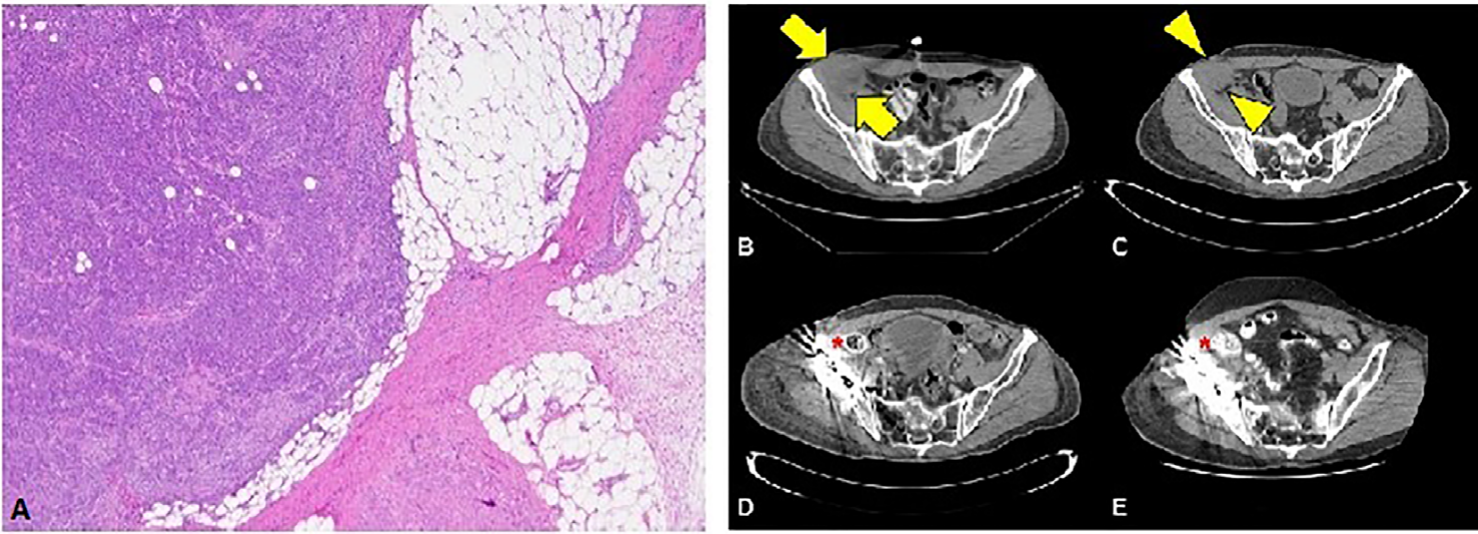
(A) Squamous cell carcinoma with perinodal extension into fat (4X magnification). (B) Cross section through the CT portion of the PET/CT prior to the initiation of immunotherapy. The largest lymph node measures 5.6 x 4.3 cm transverse (arrows). (C) Noncontrast CT of the pelvis obtained approximately 1 month after initiation of immunotherapy reveals decreasing lesion size (arrowheads) now measuring 4.2 x 3.8 cm (arrowheads). Subsequent contrast enhanced CTs at 3 months (D) and 36 months (E) after initiation of immunotherapy show no evidence of residual nodal disease.
